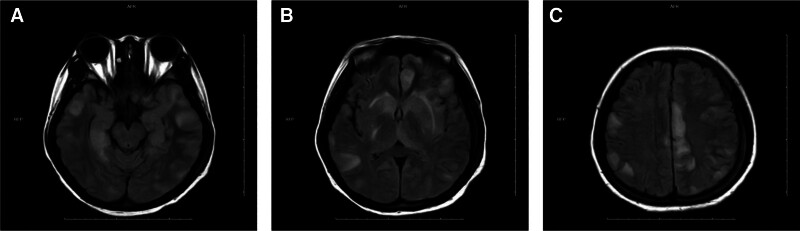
(A–C) October 27, 2023 – MRI demonstrates bilateral swelling with T2/FLAIR hyperintensity in the hippocampal formations, occipital lobes, temporal lobes, basal ganglia, frontal lobes, and parietal gyri. MRI = magnetic resonance imaging.
